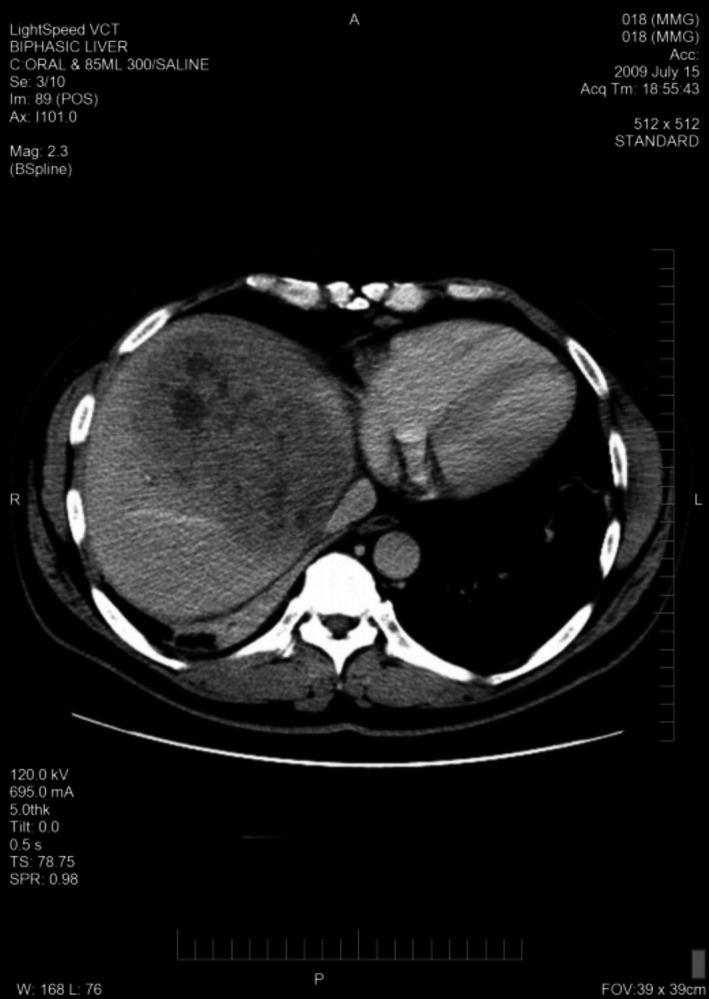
Patient 1 with Hepatocellular Carcinoma (HCC)—Baseline CT images—venous phase.
